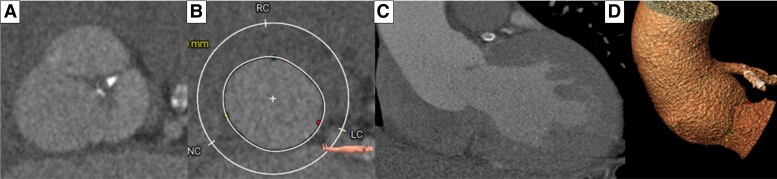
Cardiac computed tomography. (*A*) Tricuspid aortic valve with an absence of calcium in the leaflets. (*B*) A giant ring with an absence of calcium. (*C* and *D*) A dilation of the aortic root and ascending aorta can be observed.
